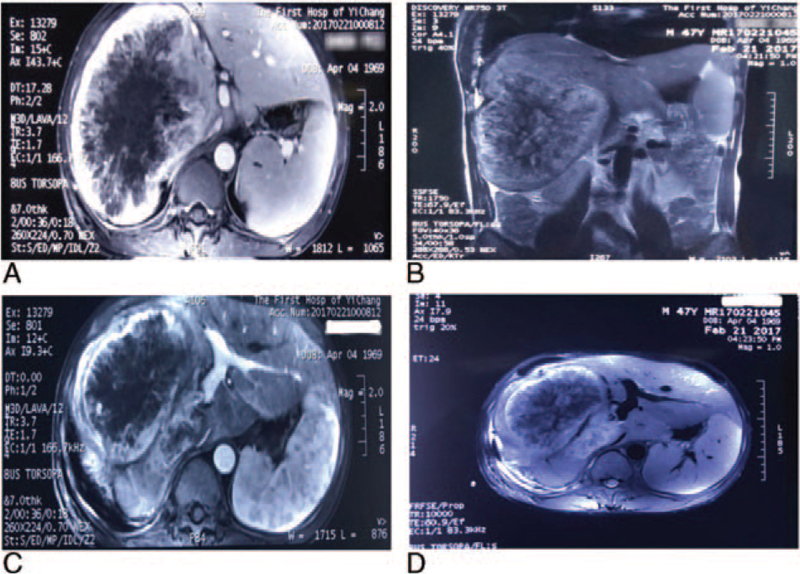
A–B: MRI of the up-abdomen showed a 19 × 15 × 13 cm lesion occupied most of the right liver. No evidence of other abdominal lesions. A MRI image in the axial plane (A, C–D) and in the coronal plane (B). MRI = magnetic resonance imaging.
